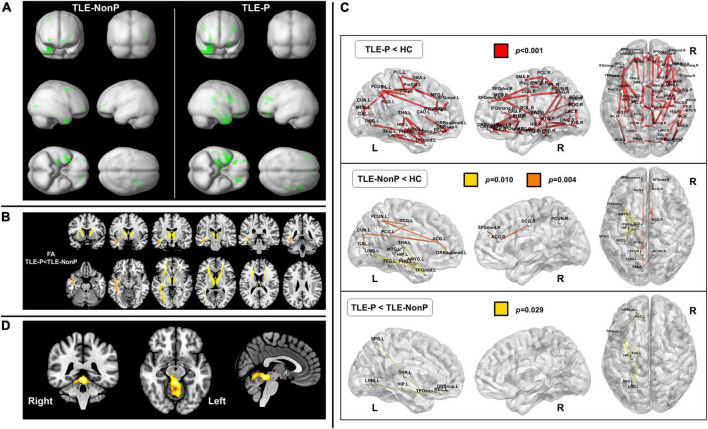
Neuroimaging findings in psychosis in epilepsy. (A) Gray matter reduction in temporal lobe epilepsy (TLE) with and without psychosis (TLE-P and TLE-NonP) compared with healthy controls, derived from structural magnetic resonance imaging (MRI). Both TLE-P and TLE-NonP showed significant volume reduction in the ipsilateral temporal and other brain areas, but there was no significant direct difference between them [Cited from Sone et al. (25)]. (B) Significant reduction in fractional anisotropy (FA) within the white matter tracts in TLE-P, derived from diffusion MRI [Cited from Sone et al. (40)]. (C) Disrupted brain white matter networks in TLE-P and TLE-NonP, derived from diffusion MRI [Cited from Sone et al. (40)]. (D) Hypermetabolic areas in TLE-P compared to TLE-NonP, derived from fluorodeoxyglucose positron emission tomography (FDG-PET) [Cited from Sone et al. (48)].
